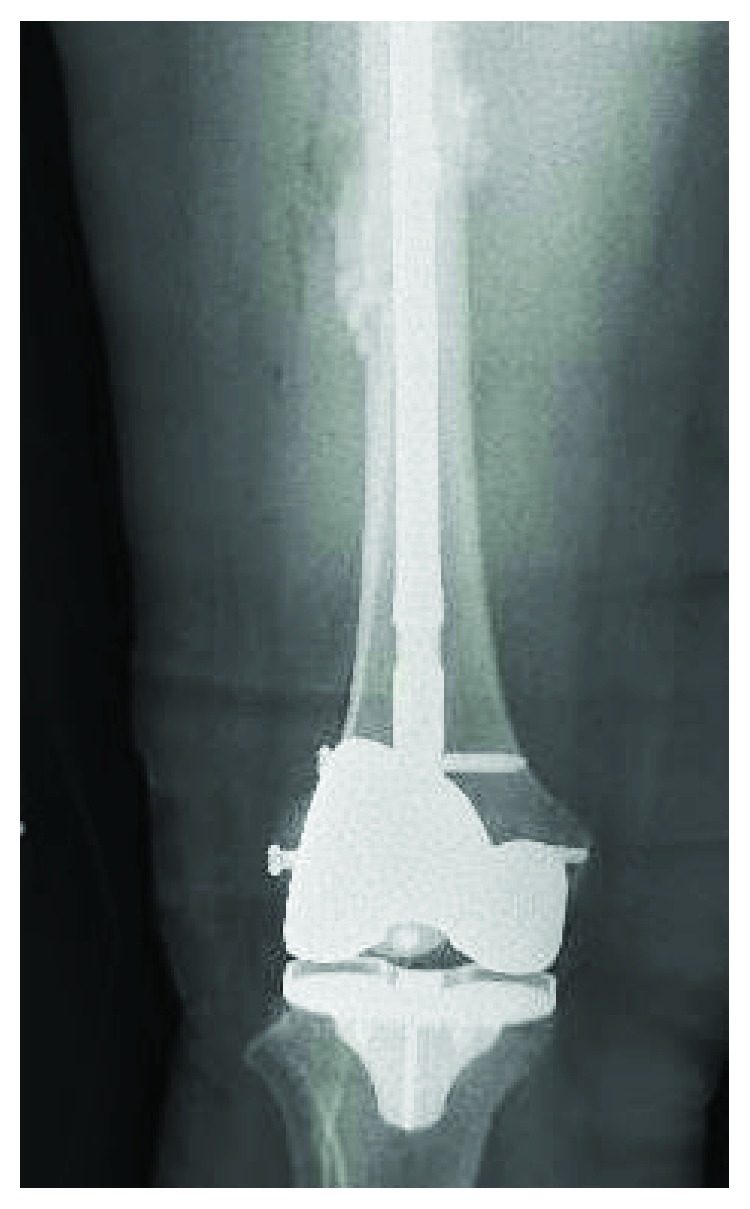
Anteroposterior radiograph of right femur with a healed fracture and stable orthopedic hardware.
